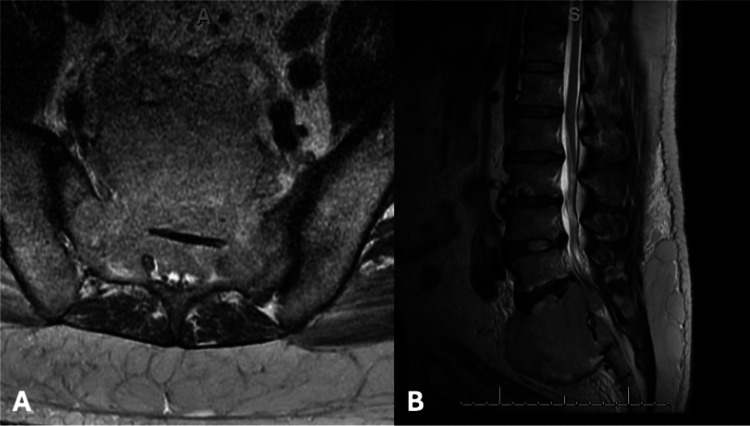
Preoperative MRI showing sacral extramedullary plasmacytoma on (A) axial and (B) sagittal T2-weighted images
